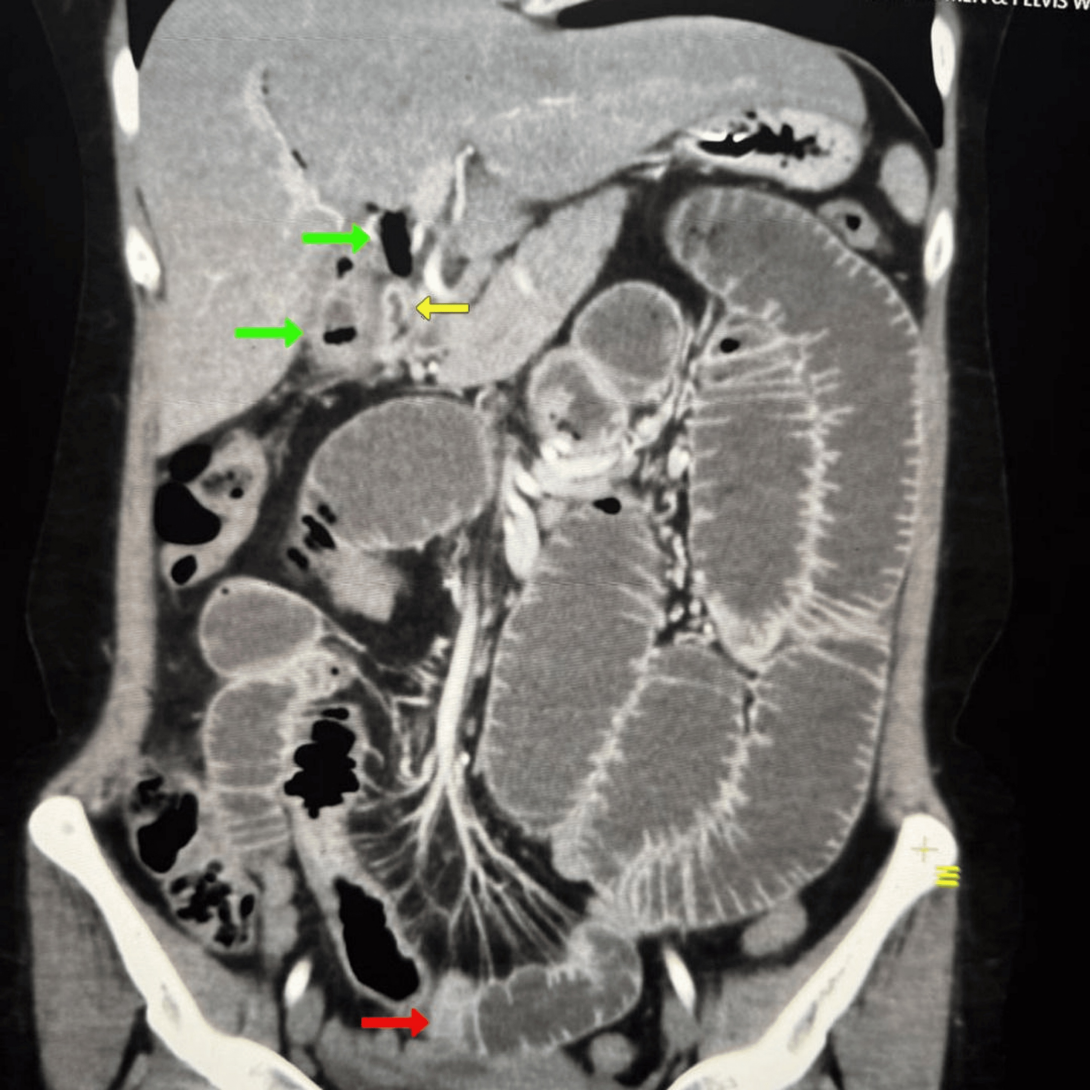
Contrast-enhanced CT demonstrating small bowel obstruction, pneumobilia (green arrow), and a 3x3 cm calcified intraluminal density (gallstone red arrow) in the distal ileum, along with cholecystoduodenal fistula (yellow arrow)
